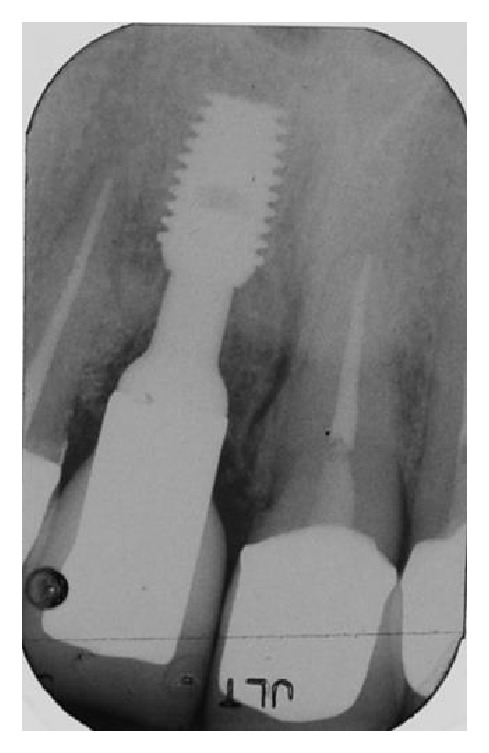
Control X-ray at the end of therapy.
